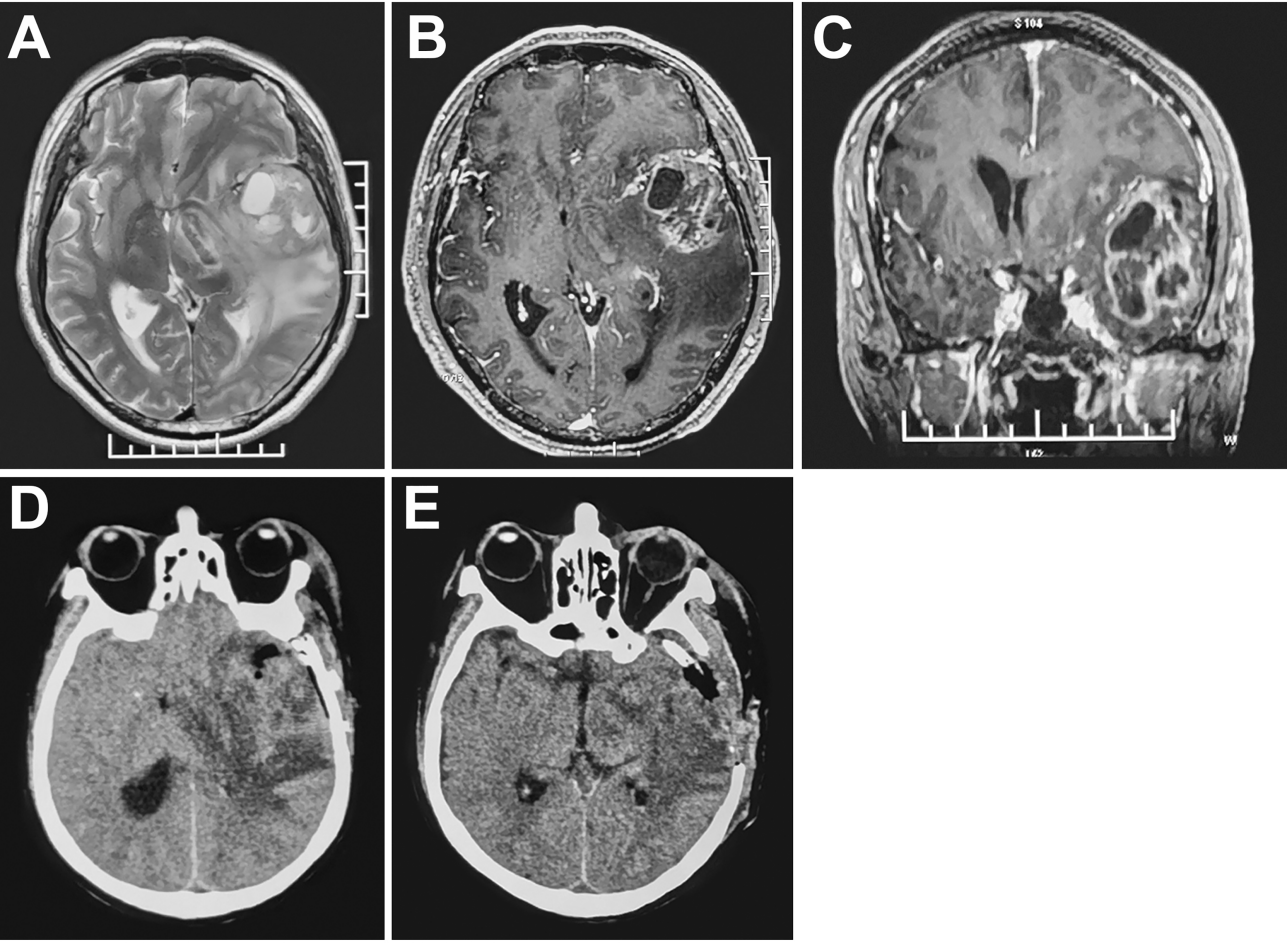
MRI (A-C) high-grade glioma with marked peritumoral edema in the left temporal lobe. CT (D) subtotal tumor resection, obvious midline shift and increased peritumoral edema 48 hours postoperatively. CT (E) Resection of residual tumor and decompressive craniectomy.
